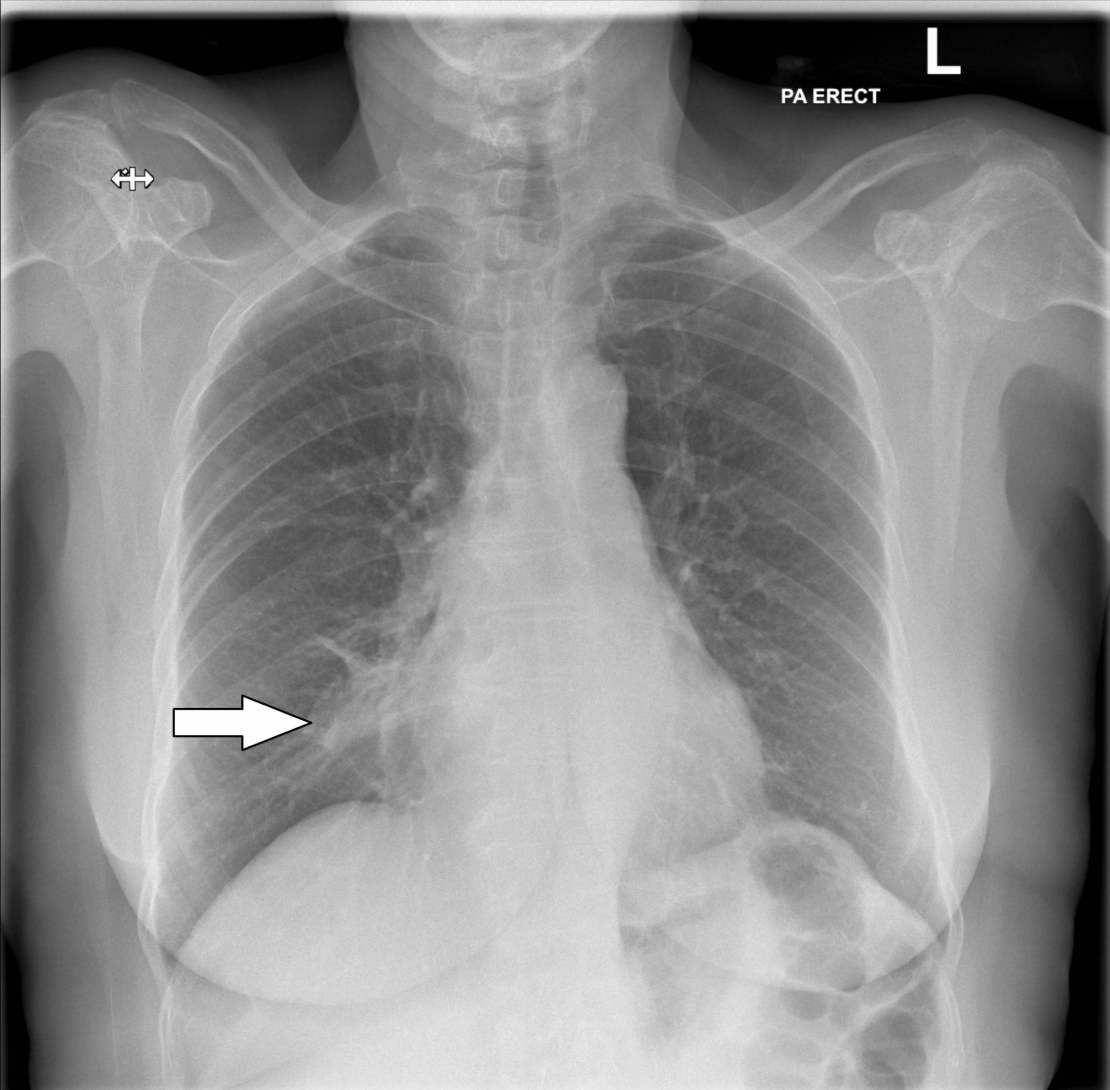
Initial CXR showing right middle-lobe consolidation CXR: chest x-ray
